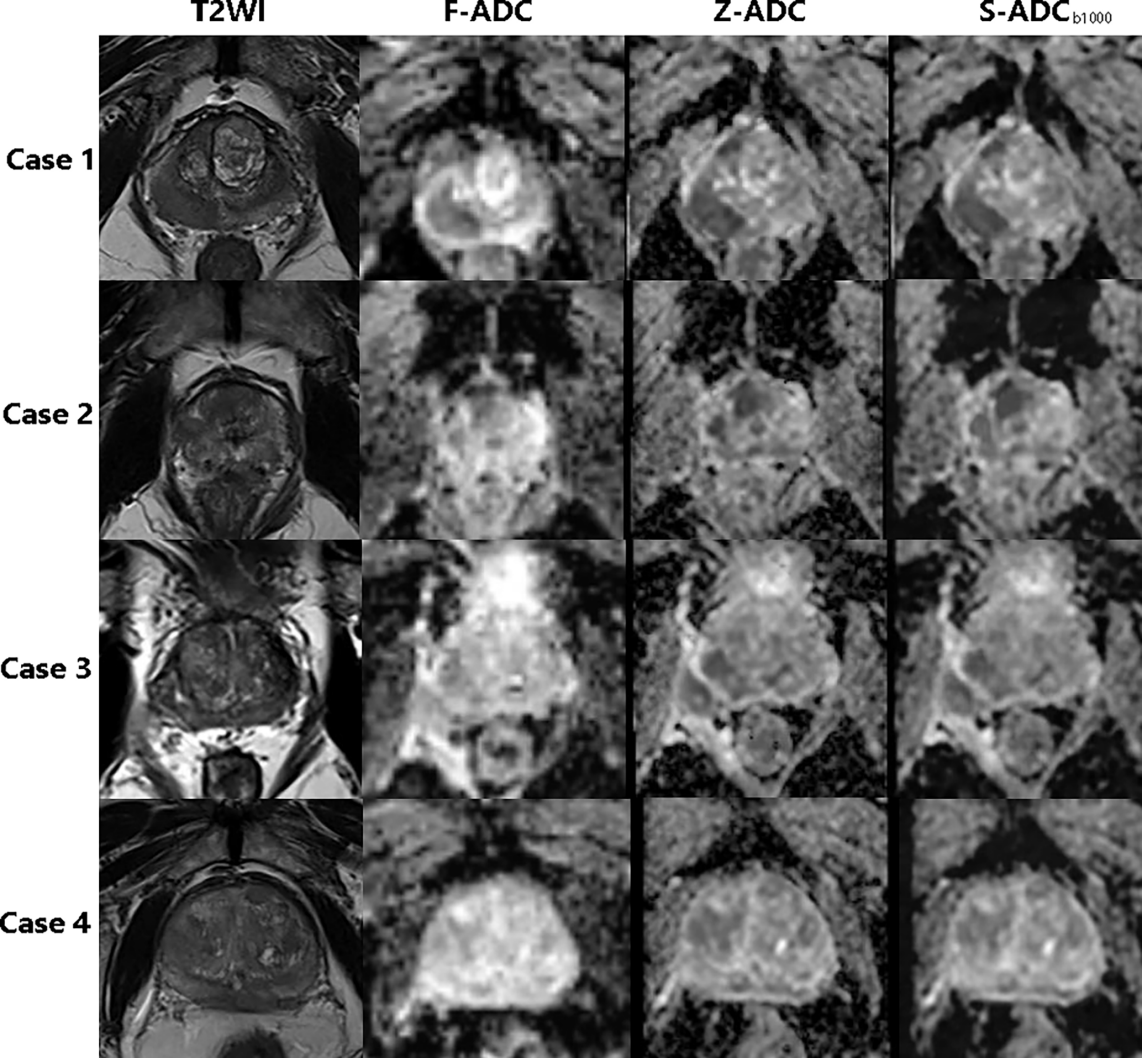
The T2-weighted image, f-ADC, z-ADC, and s-ADC_b1000_ of four different patients. Case 1: An 85-year-old man with prostate cancer in the right peripheral zone and an initial prostate-specific antigen level of 0.157 ng/ml. Case 2: An 85-year-old man with prostate cancer in the central zone and an initial prostate-specific antigen level of 21.44 ng/ml. Case 3: A 67-year-old man with an inflammatory nodule in the right peripheral zone and an initial prostate-specific antigen level of 14.37 ng/ml. Case 4: A 77-year-old man with prostate cancer in the central zone and an initial prostate-specific antigen level of 56.62 ng/ml.
